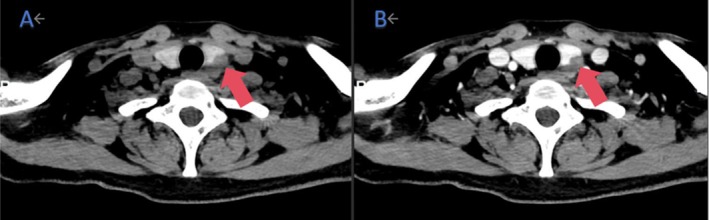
The left lobe of the thyroid gland showed a slightly low‐density shadow, and the CT value was about 48 HU on plain scan (A), with moderate enhancement in the arterial phase, the CT value about 82 HU (B) in March 2023.
